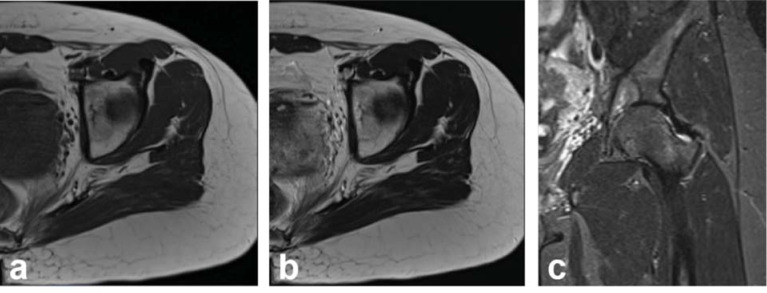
Changes in MRI images after 11 months of antifungal therapy. (a) Axial image of T1-weighted image. (b) Axial image of T2-weighted image. (c) Coronal image of T2-weighted image. MRI showed a reduction in the size of the lesion, but there was still some bone defect in the left acetabulum.
